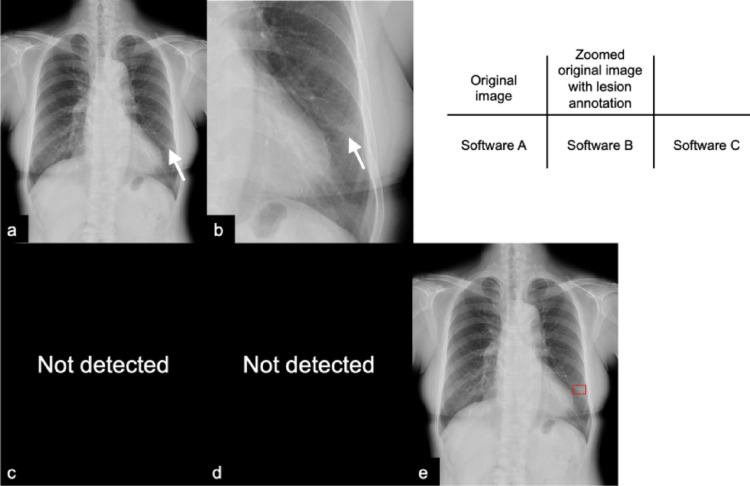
Representative case: lesion missed by first reader but detected by one AI models (66-year-old male, BI = 0). a and b. Chest radiograph showing a nodule in the left lower lung (arrow) identified by the second reader. c–e the lesion was not detected by the first reader; in the AI outputs, Software A (c) and Software B (d) did not detect the lesion at the target site, whereas Software C (e) correctly localized the lesion (red bounding boxes). The lesion’s visibility score was 1 (very subtle)
